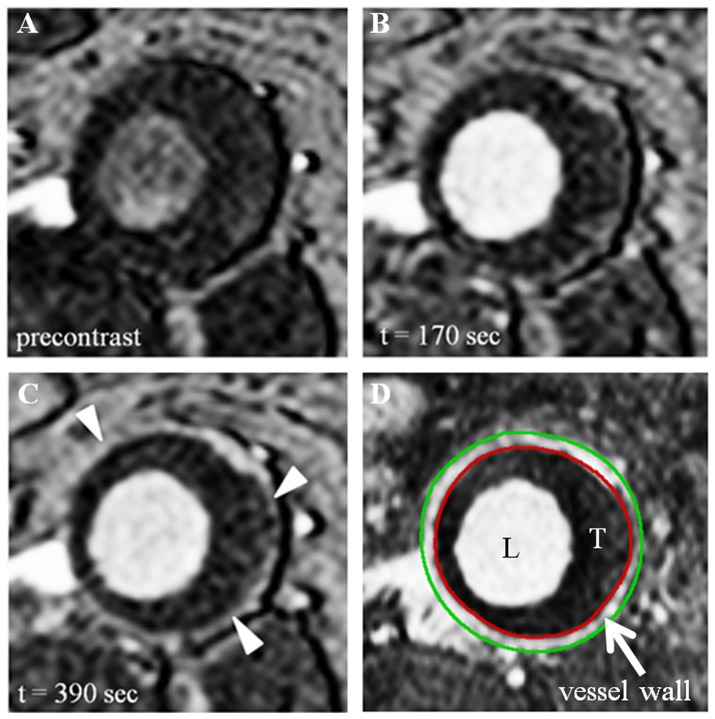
Dynamic contrast-enhanced MR images of the AAA vessel wall. (A, B and C) T1weighted images from a dynamic serie at the level of the maximal diameter acquired respectively before contrast injection, 3 and 6.5 minutes after contrast injection. Vessel wall enhancement already occurs in (B) but is clearly visible in (C) (indicated by arrow heads). (D) Contrast-enhanced T1weighted anatomical image at the same level as in (A,B and C) with drawn inner (red circle) and outer (green circle) vessel wall ROI.
